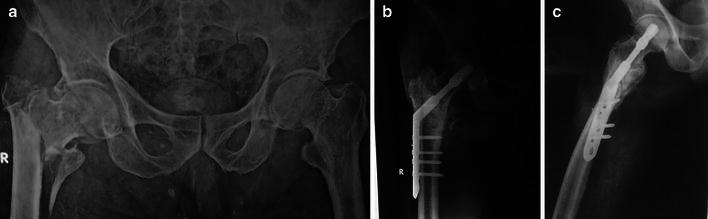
a Preoperative anteroposterior radiograph in a 75-year-old male showing 31A2.3 pertrochanteric fracture. b Follow-up anteroposterior radiograph of the same patient showing union. c Follow-up lateral radiograph of the same patient showing union
